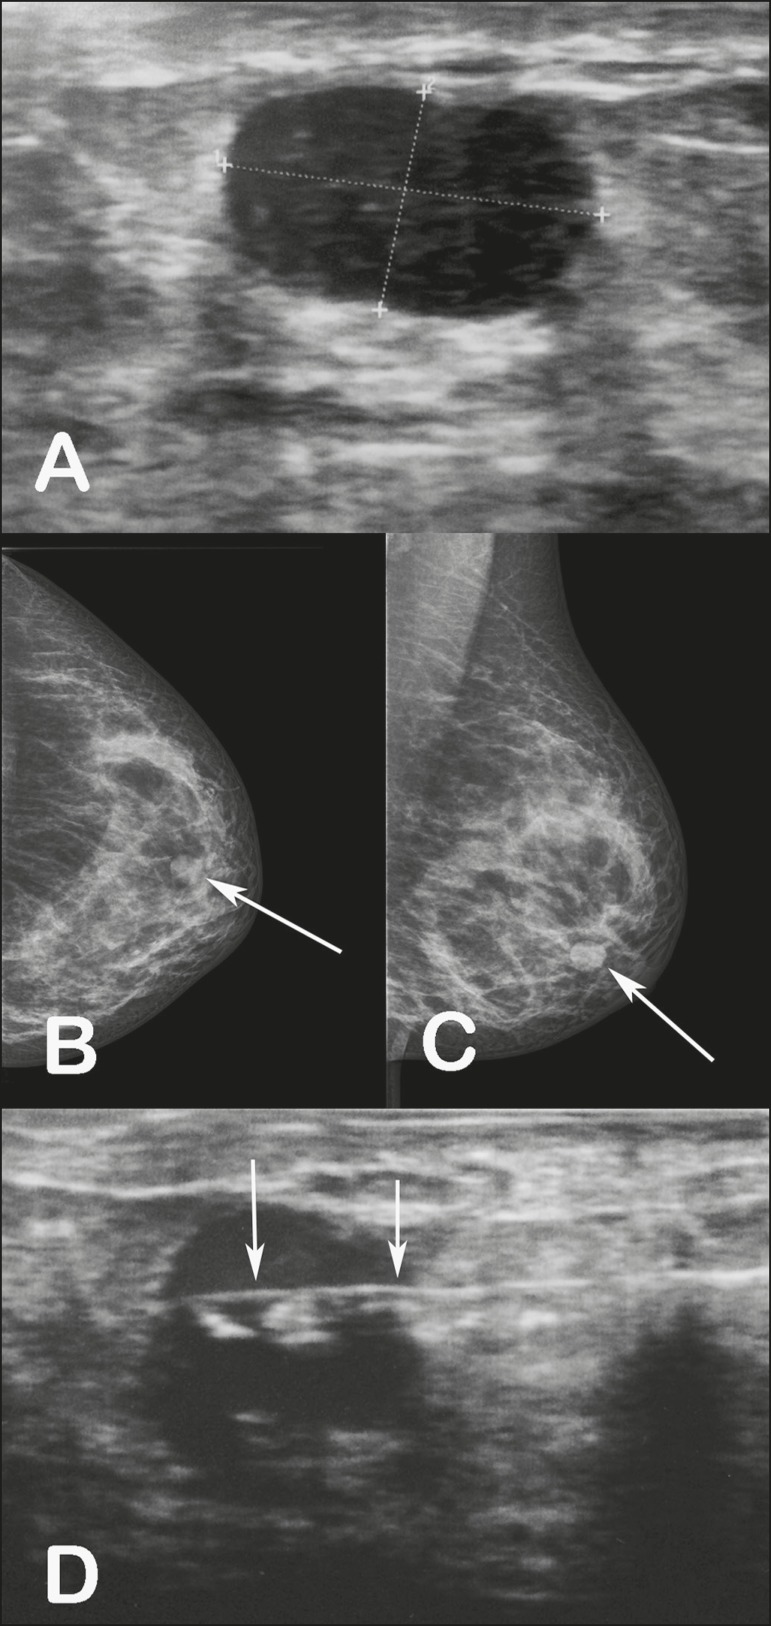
A: Ultrasound showing a well-circumscribed, hypoechoic nodule, measuring 12
